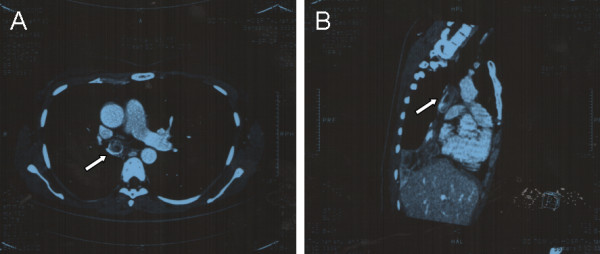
Chest computed tomography showed an intraluminal tumor shadow on the bifurcation of the right main bronchus, involving to the carina, blocking about 90% of the right main bronchus lumen (A, Cross section; B, Sagittal plane).
